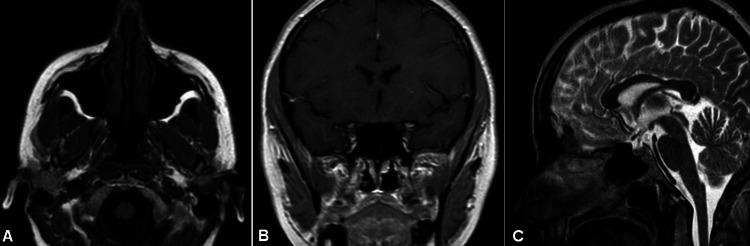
Sinus MRI Sinus MRI following endoscopic resection revealed normal findings. A. Axial (T1W1 sequence), B. Coronal (T1W1 sequence), C. Sagittal (T2W1 sequence)
